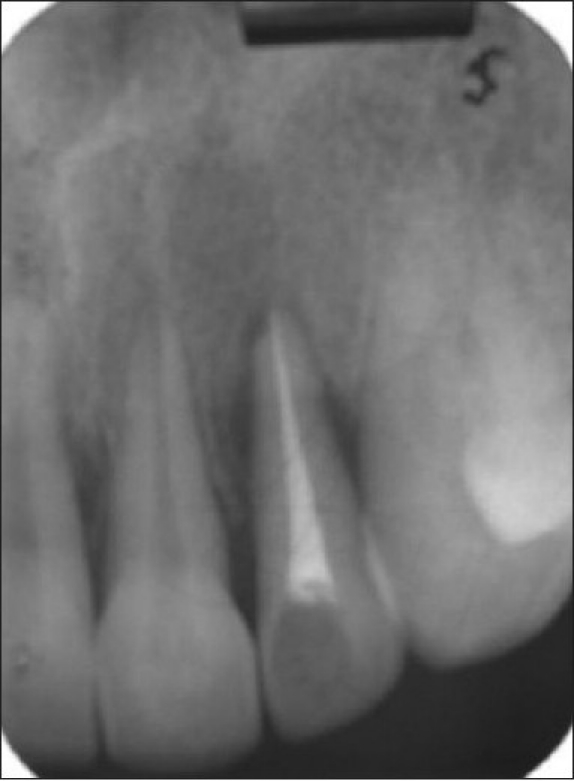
Post-treatment radiograph after periodontal flap surgery and intrusive orthodontic therapy
